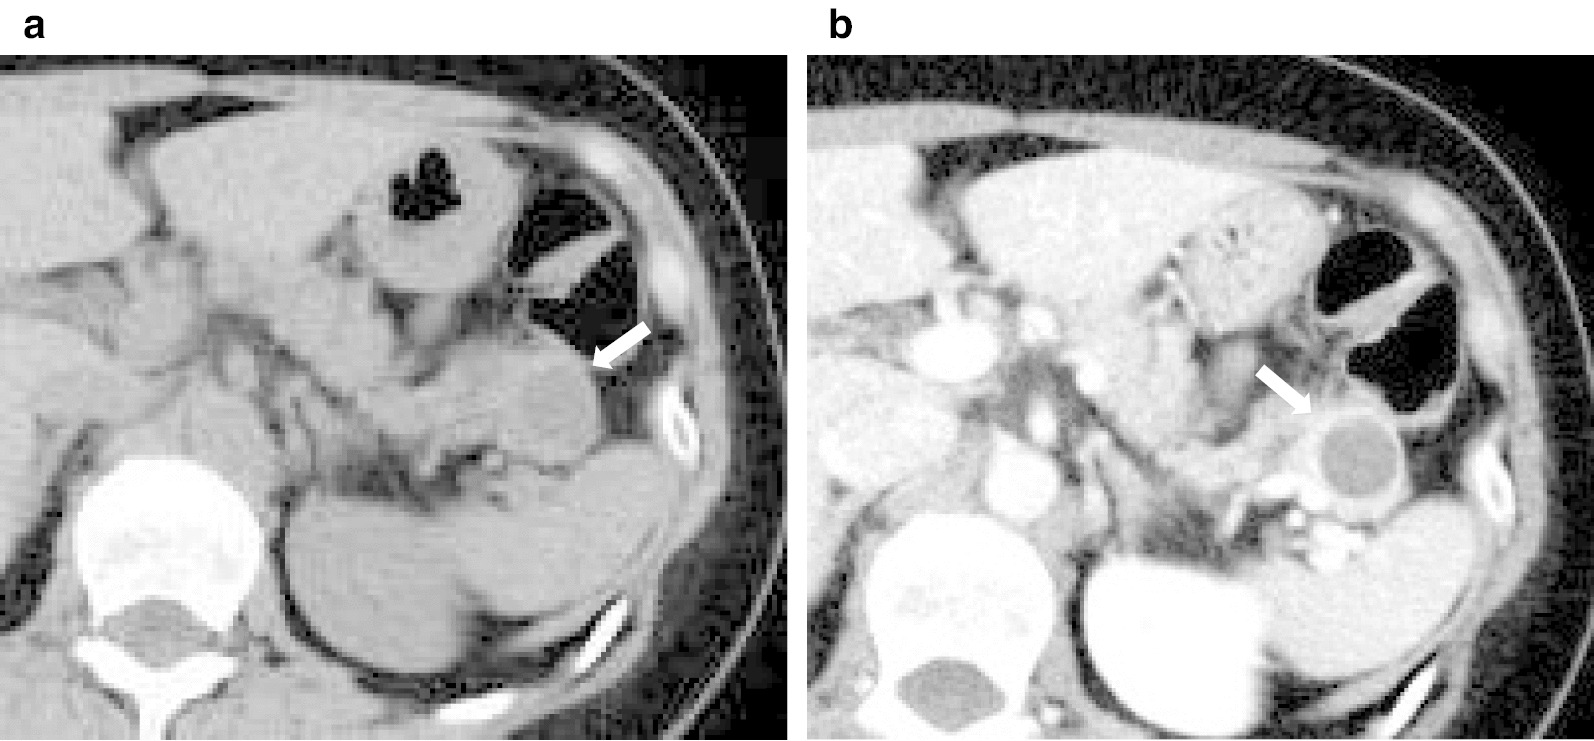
The contrast-enhanced abdominal computed tomography (CT) scan confirmed a unilocular cystic lesion (arrows) measuring 16 mm in size in the pancreatic tail. The wall of the cyst appeared to be enhanced. (a plain, b portal phase)
